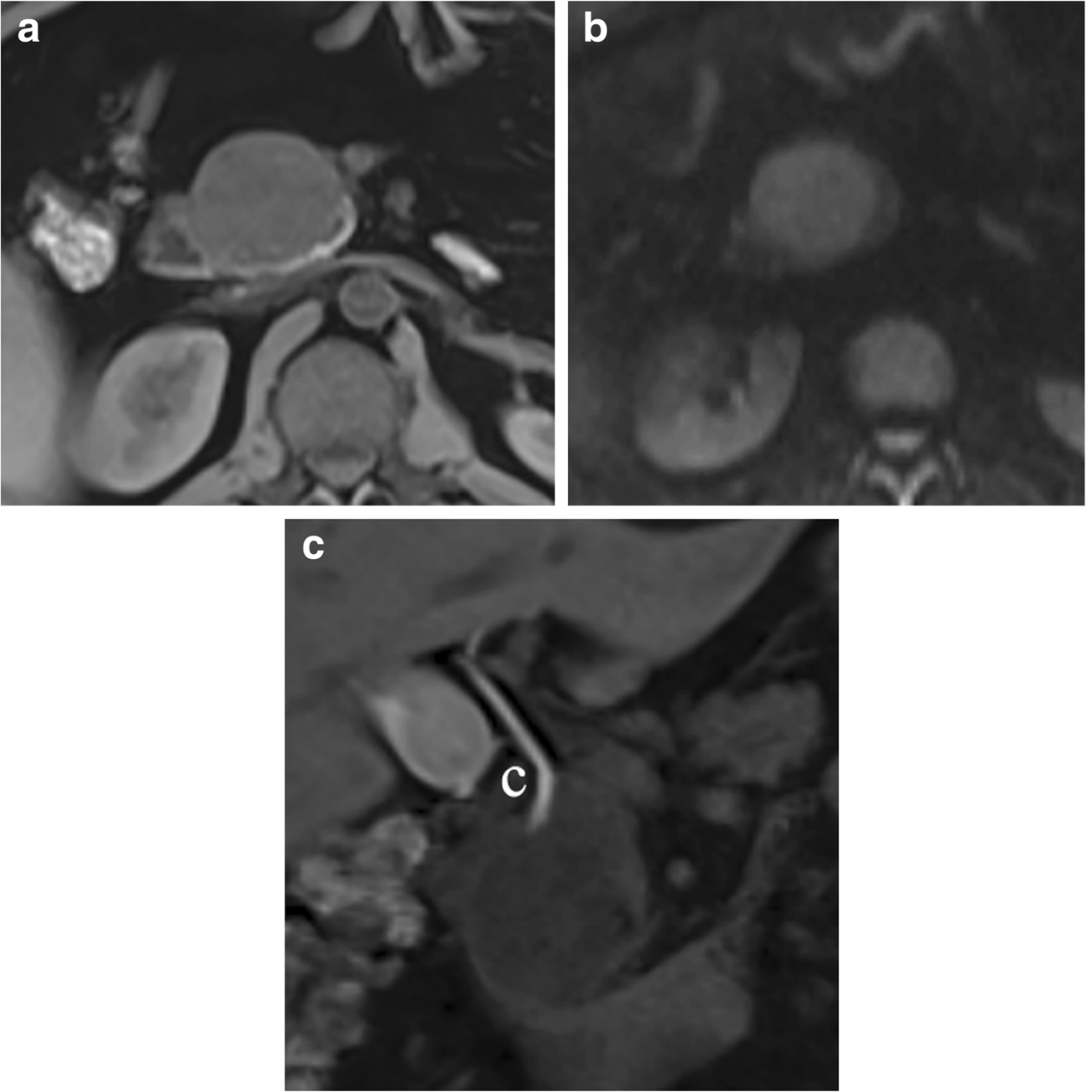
Capsulated NEN. MRI study: the pancreatic head lesion is slightly hypointense on T1-weighted fat-saturated axial images (a) and presents diffusion restriction (b) on DWI (*b* = 800). In the late hepatospecific phase (c) with contrast medium (Gd-BOPTA), the common bile duct (*C*) is clearly visible and not dilated, since it is displaced but not compressed by the pancreatic head mass
